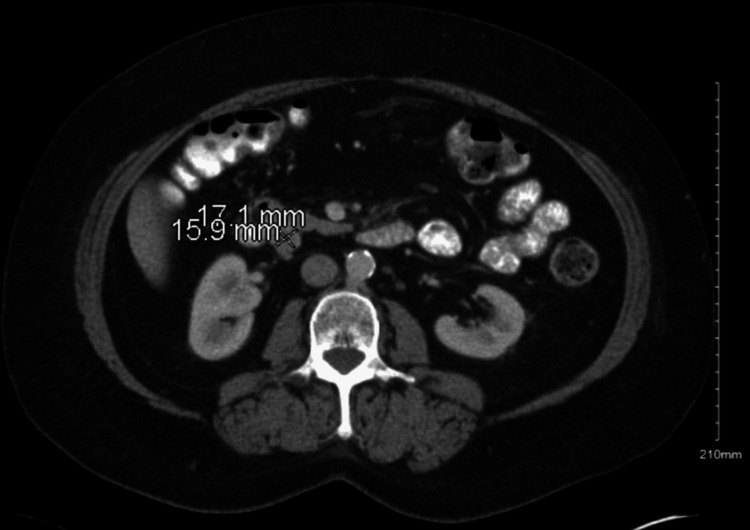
Computed tomography of the abdomen showing a mass in the posterior pancreaticoduodenal groove
